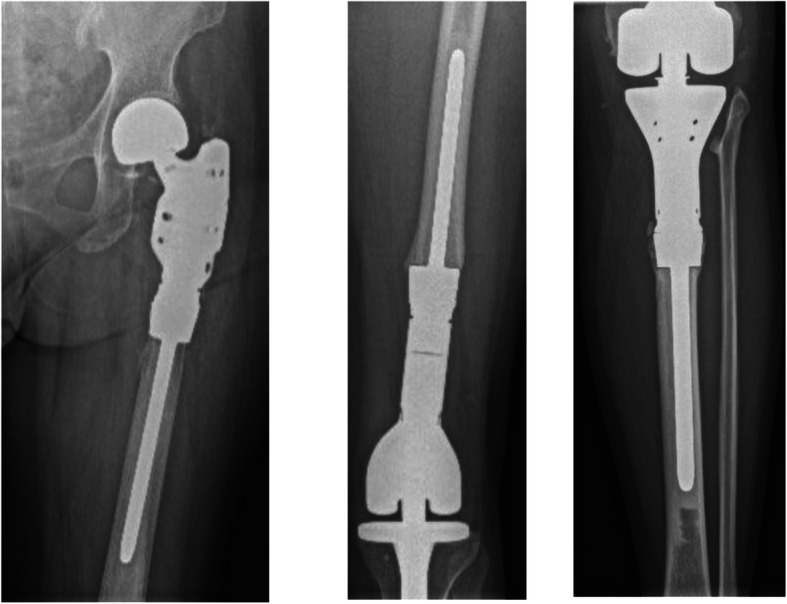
Radiographs of proximal femur tumor arthroplasty, Cemented Zimmer® Segmental stem (left). Radiographs of distal femur arthroplasty, Cemented Zimmer® Segmental stem (middle). Radiographs of proximal tibia arthroplasty, Cemented Zimmer® Segmental stem (right)
